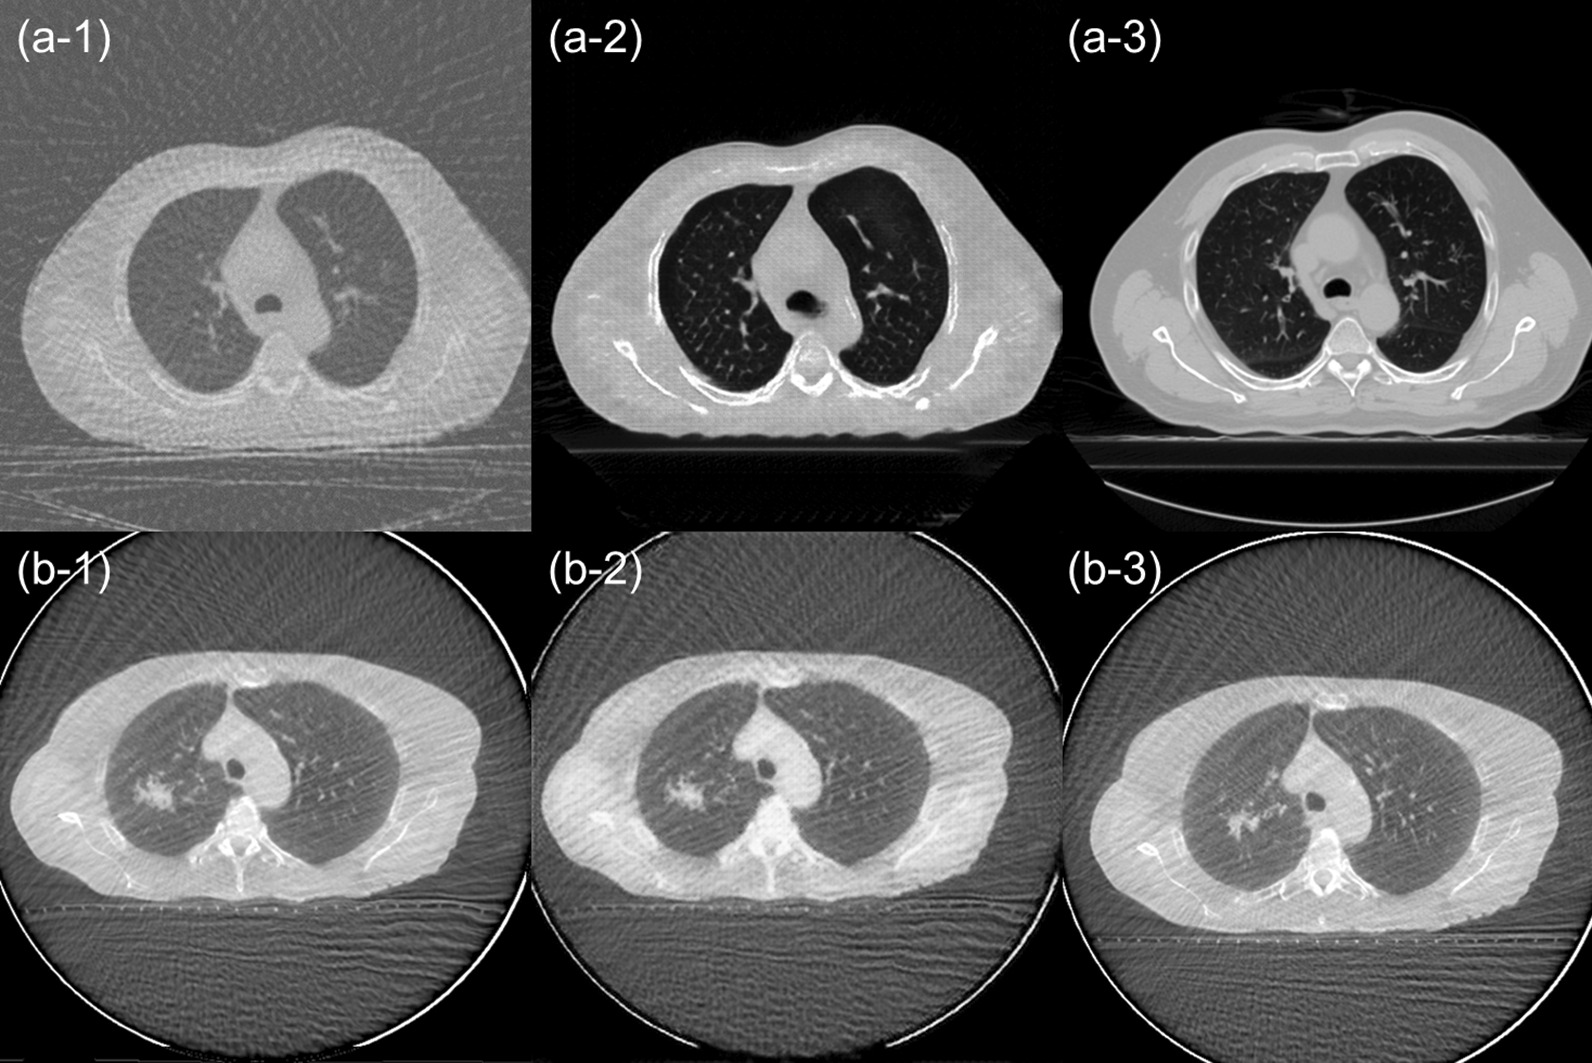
Results of the image quality test (a) and image deformation test (b). All images are shown with the same window width and levels. a-1 Degraded 4D-MSCT image, a-2 generated image, and a-3 original 4D-MSCT image. b-1 Original 4D-CBCT image in maximum exhalation, b-2 generated image, and b-3 4D-CBCT image in maximum intake
